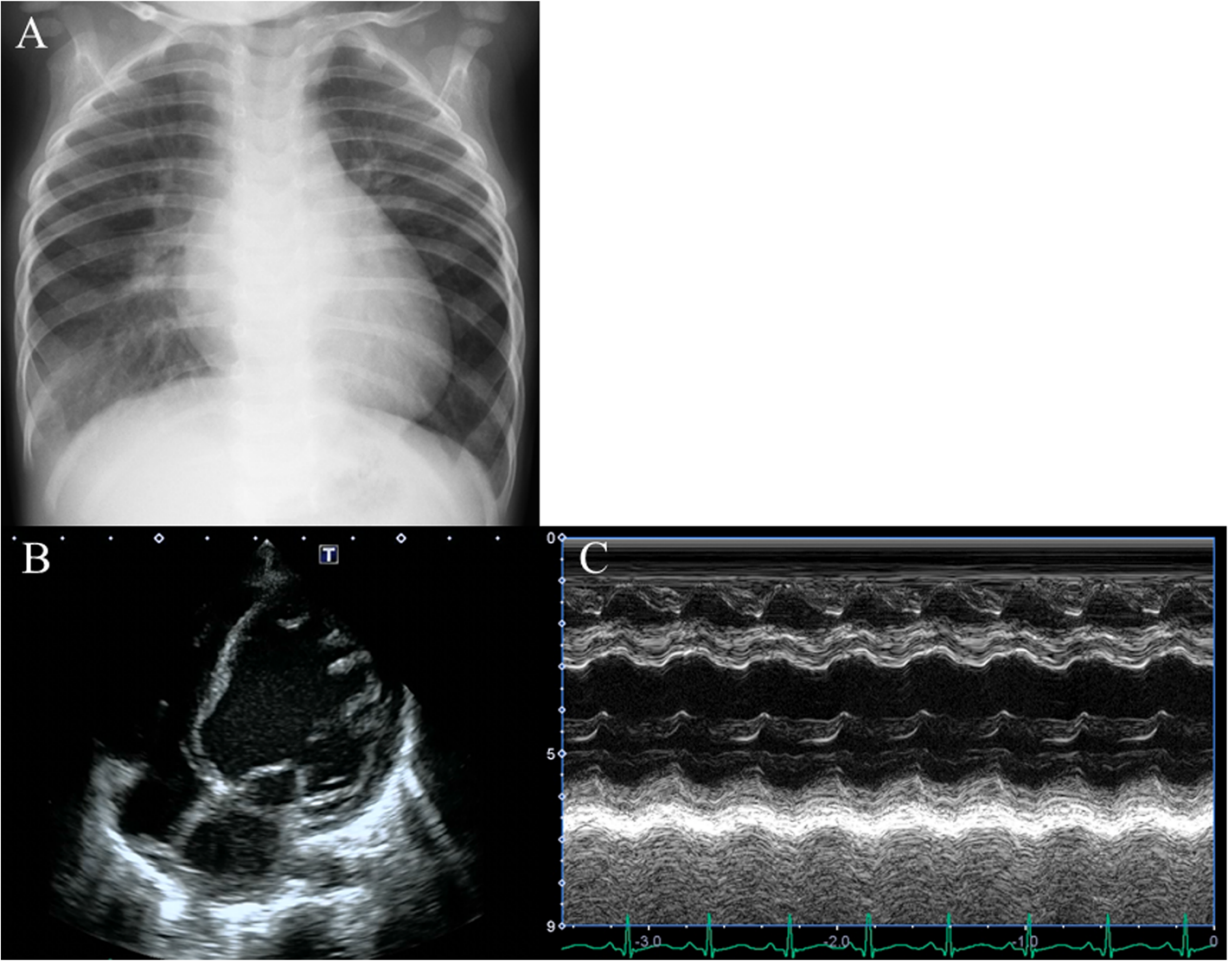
Images taken at admission. a Chest X-ray. b, c Four-chamber and M-mode view of echocardiography, respectively
